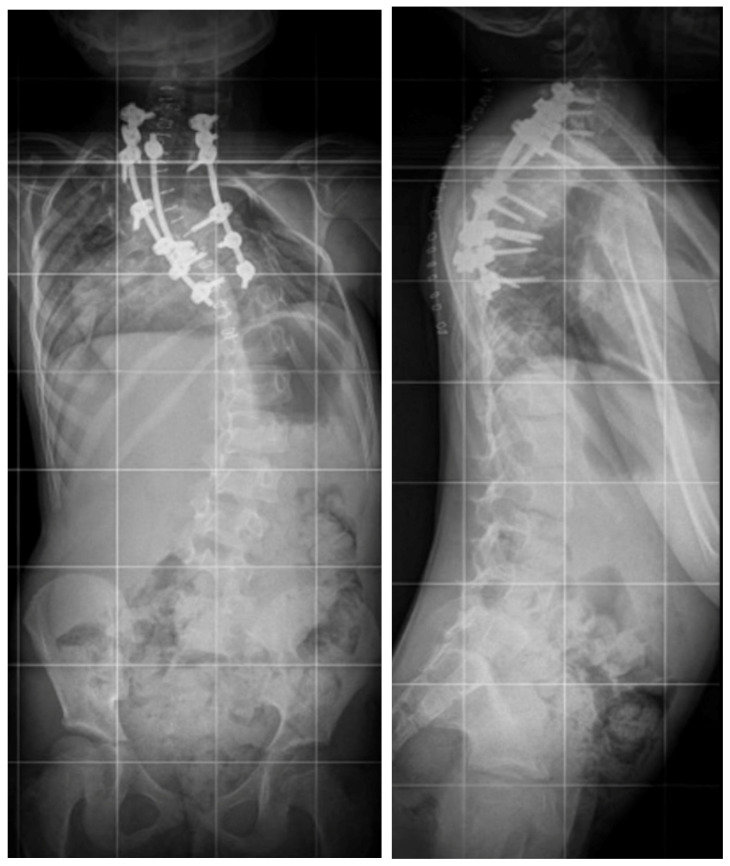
Standing X-rays of the five-year-old girl after surgical treatment via the posterior approach and VCR T3–T4.
